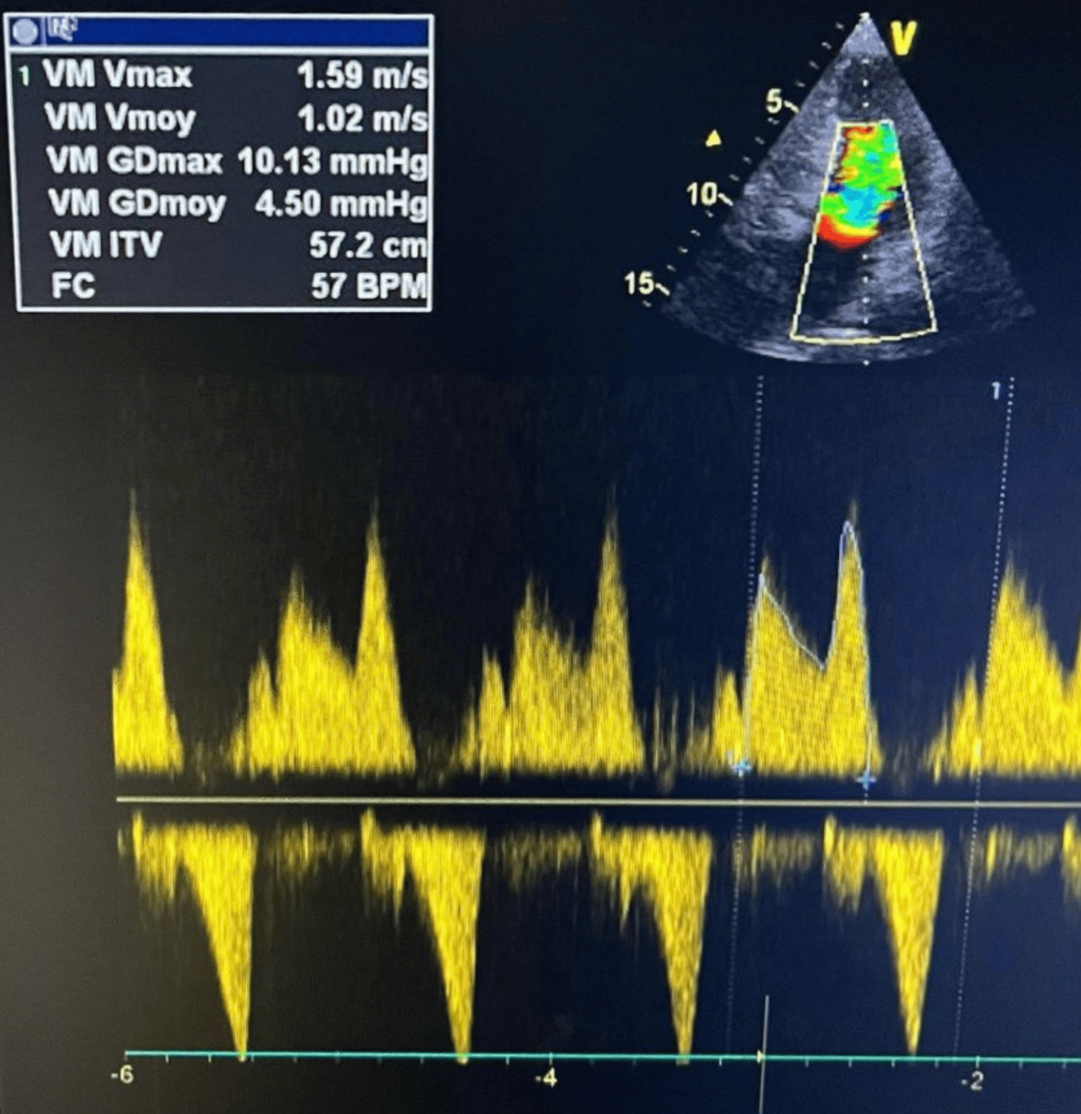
Continuous-wave Doppler revealing a systolic mid-cavity “saber-shaped” signal. Continuous-wave Doppler tracing recorded from the apical window showing a characteristic systolic mid-ventricular “saber-shaped” flow signal, reflecting a high-velocity gradient within the mid-cavity. This pattern is typical of mid-ventricular obstruction in hypertrophic cardiomyopathy.
